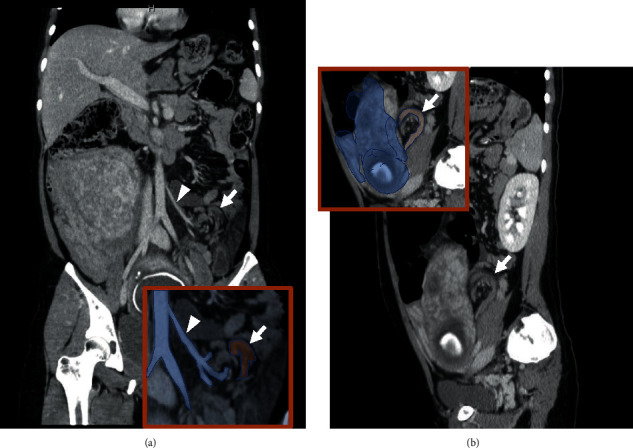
(a) Abdominal contrast-enhanced computed tomography (CT) (coronal view) with sigmoidal torsion (arrow) at the periphery of the inferior mesenteric artery (arrowhead). (b) Abdominal contrast-enhanced CT (sagittal view)—a portion of the torsion is located on the dorsal side of the uterus (arrow).
